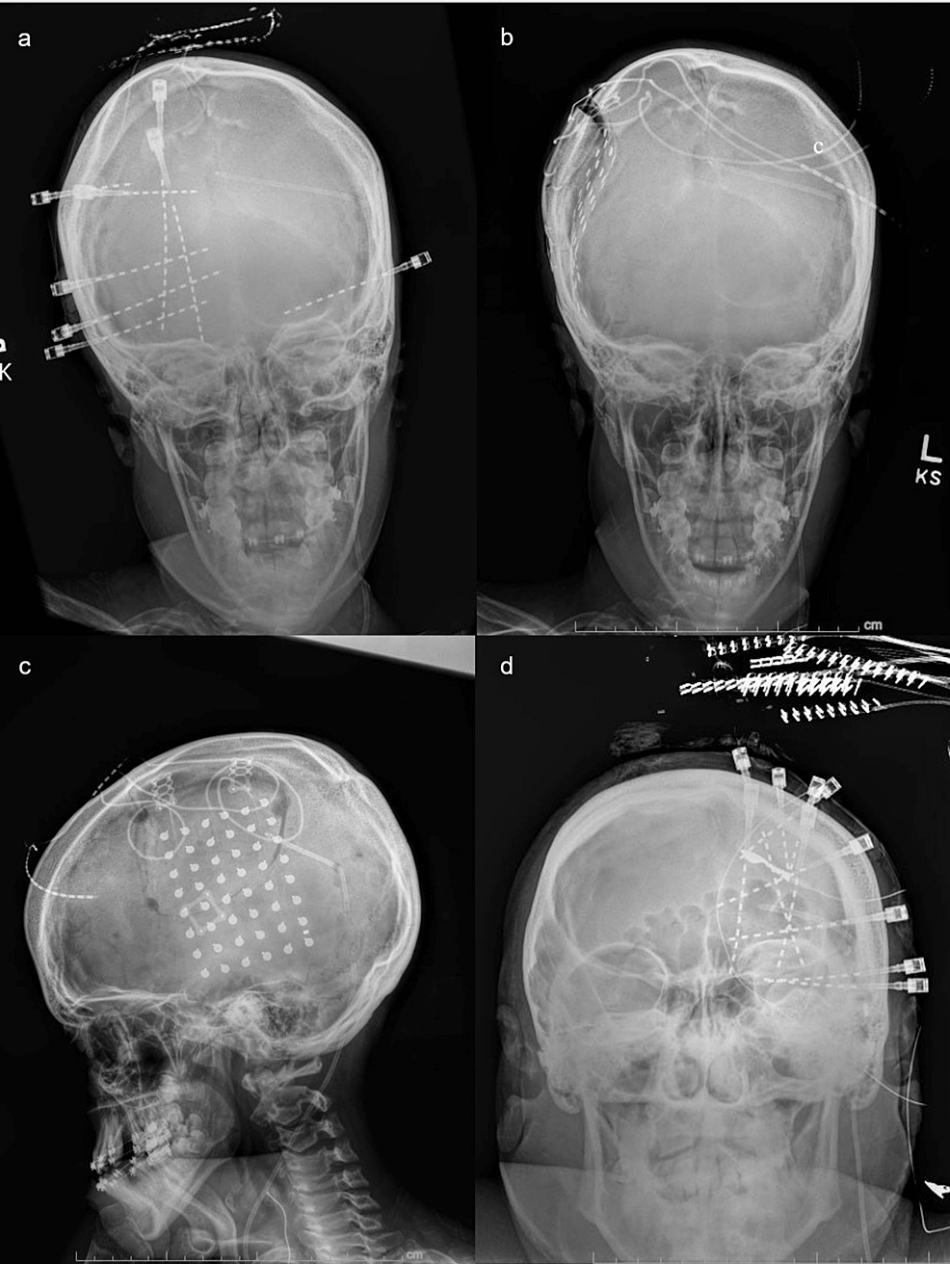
X-rays showing the location of invasive intracranial monitoring devices in three cases of shunted hydrocephalus. a. Patient 1 (invasive intracranial monitoring procedure 1 – SEEG implantation), eight depth electrodes placed in bilateral hemispheres with shunt in the left ventricle. b. Patient 1 (invasive intracranial monitoring procedure 2 – intracranial grid implantation), anteroposterior X-ray showing 48 contact subdural grids overlying the right frontoparietal area with left ventricular shunt. c. Patient 1 (invasive intracranial monitoring procedure 2 – intracranial grid implantation), lateral X-ray showing 28 contact subdural grids overlying the right frontoparietal area with left ventricular shunt d. Patient 2 (SEEG implantation), 10 depth electrodes positioned in the left hemisphere with left ventricular shunt in place. SEEG, stereotactic electroencephalogram
